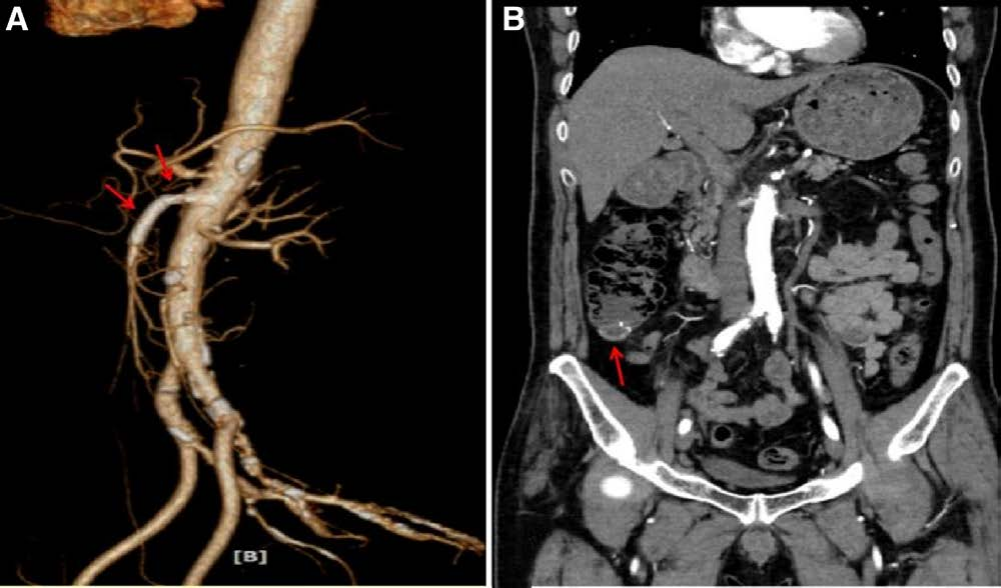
(A) Abdominal CTA reexamination showing that 9 months after operation, the stents at the opening of superior mesenteric artery were in place and the blood flow was smooth. (B) No intestinal abnormality. CTA = computed tomography angiography.
